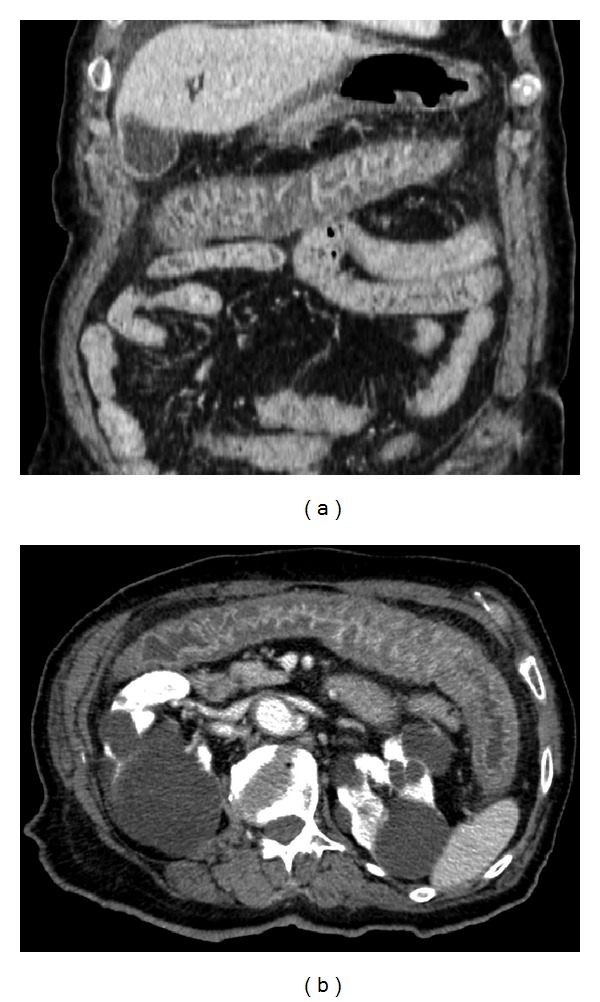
MPR CT images documenting parietal thickening and venous engorgement of the transverse colon (a, b).
